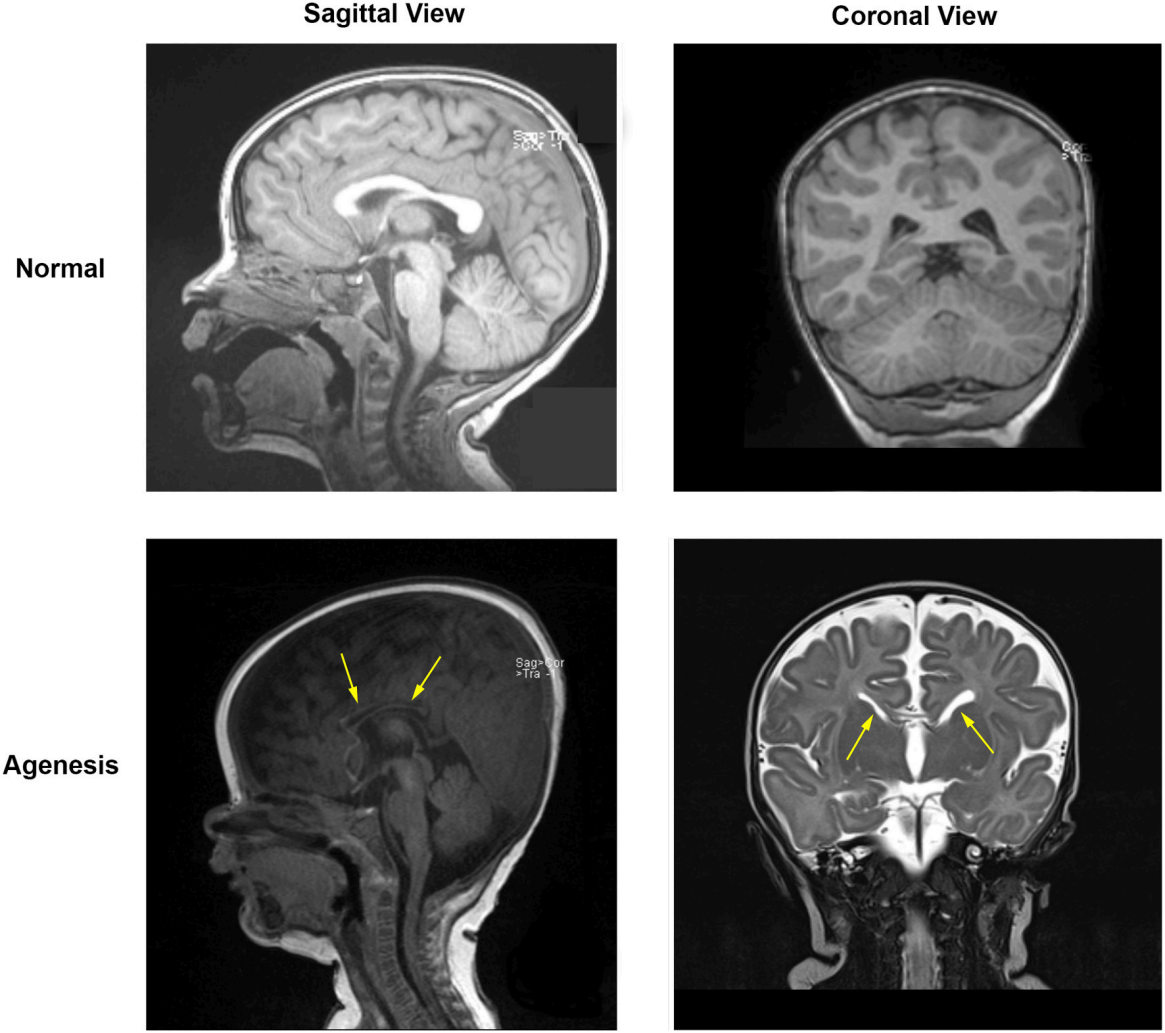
MRI data for a normal subject (top) and for a subject with partial agenesis (bottom). A rift in the corpus callosum (arrows) is clearly visible in the coronal MRI image for the patient with agenesis, which is not seen in the normal subject. White matter tracts connect below and through the cortex in the normal subject, but do not do so in the patient with agenesis.
